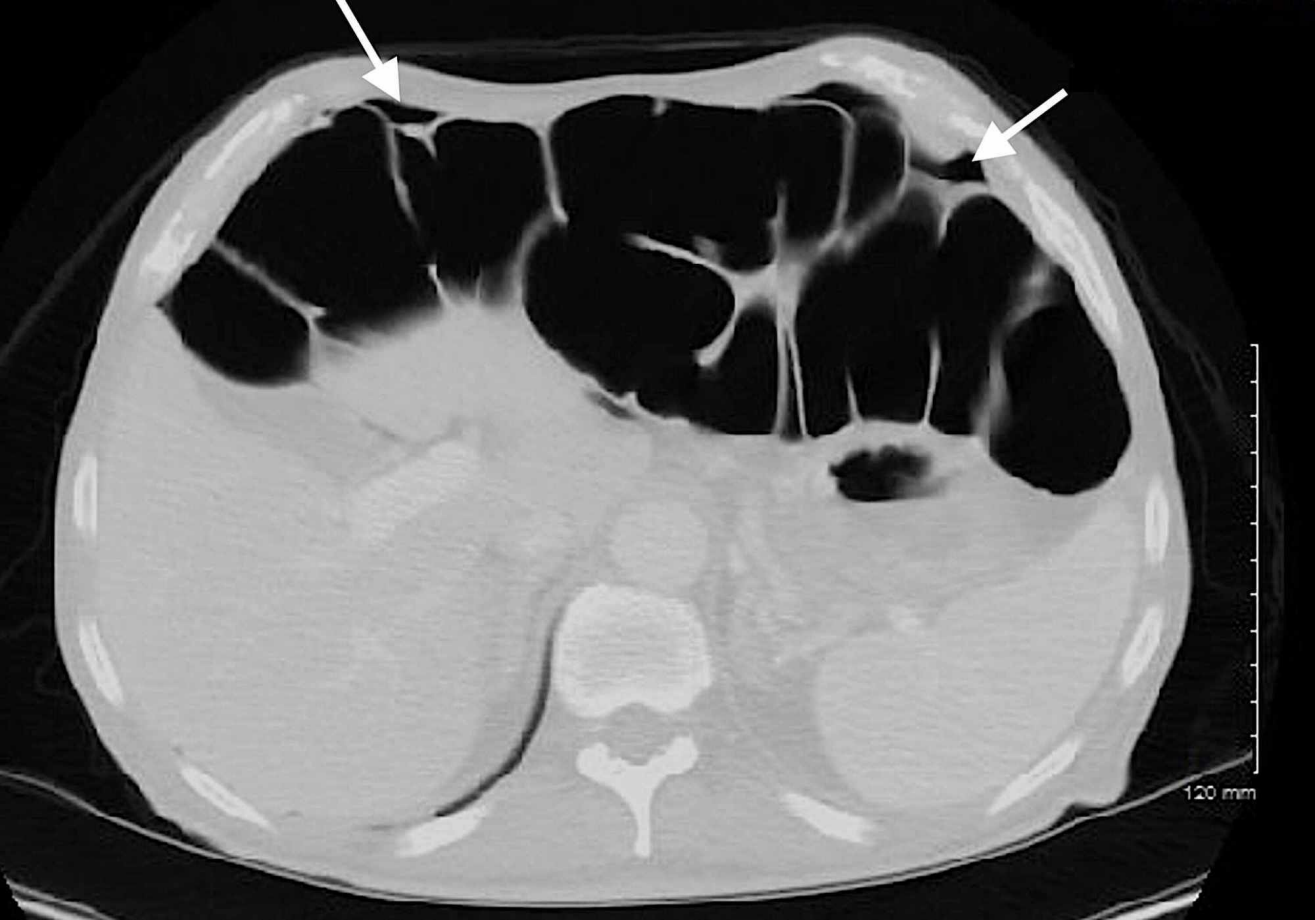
CT of the abdomen/pelvis demonstrating intraperitoneal free air (white arrows).
